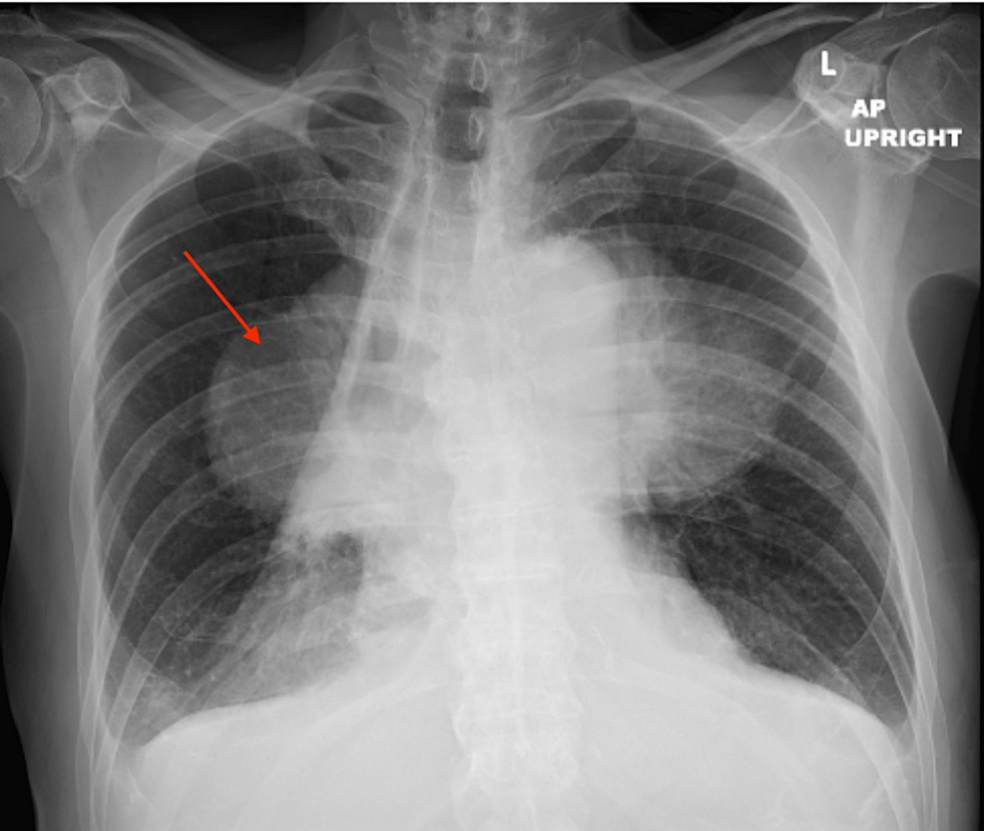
Initial chest X-ray A large ovoid opacity extending across bilateral midlungs and mediastinum is indicated by the red arrow, indicating a possible mass.
